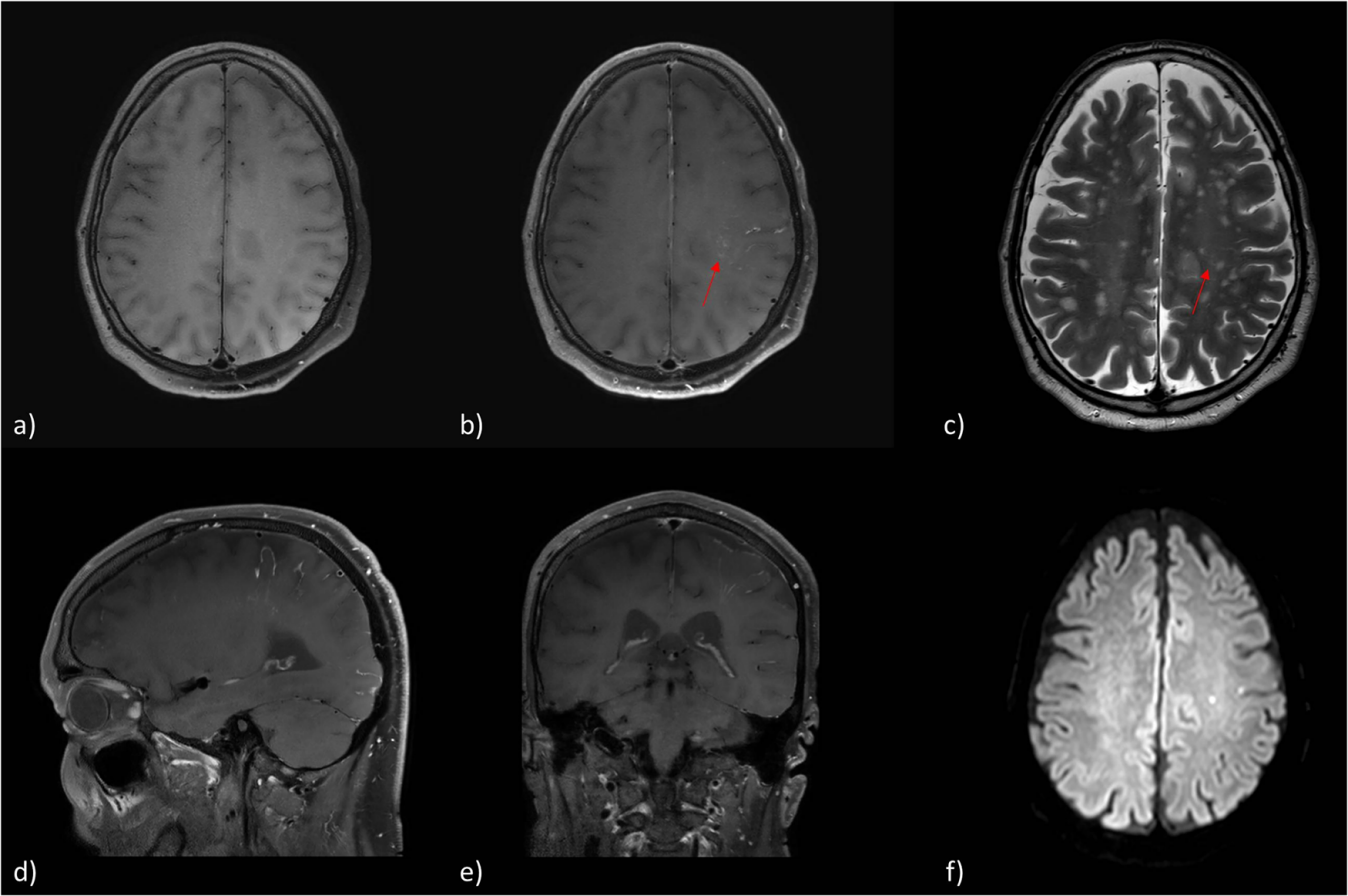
MRI examination 24 h after digital subtraction angiography for aneurysm embolization of the left MCA bifurcation with a Woven EndoBridge device. a Axial reconstruction of native three-dimensional T1-weighted sequence before GBCA administration. b Axial reconstruction of three-dimensional T1-weighted sequence 5 min after GBCA administration showing a pial and parenchymal enhancement (red arrow) in the left perirolandic region. The pial enhancement is limited to the immediate surrounding of the cerebral vasculature; c axial T2w sequence on the same level depicting the course of the perivascular spaces of the centrum semiovale (red arrow); sagittal (d) and coronal (e) reconstruction of the three-dimensional T1-weighted sequence 5 min after GBCA administration. f DWI sequence showing the only two present microembolisms in the left hemisphere, including the precentral gyrus. MCA, Middle cerebral artery; DWI, Diffusion-weighted imaging; GBCA, Gadolinium-based contrast agent; MRI, Magnetic resonance imaging
